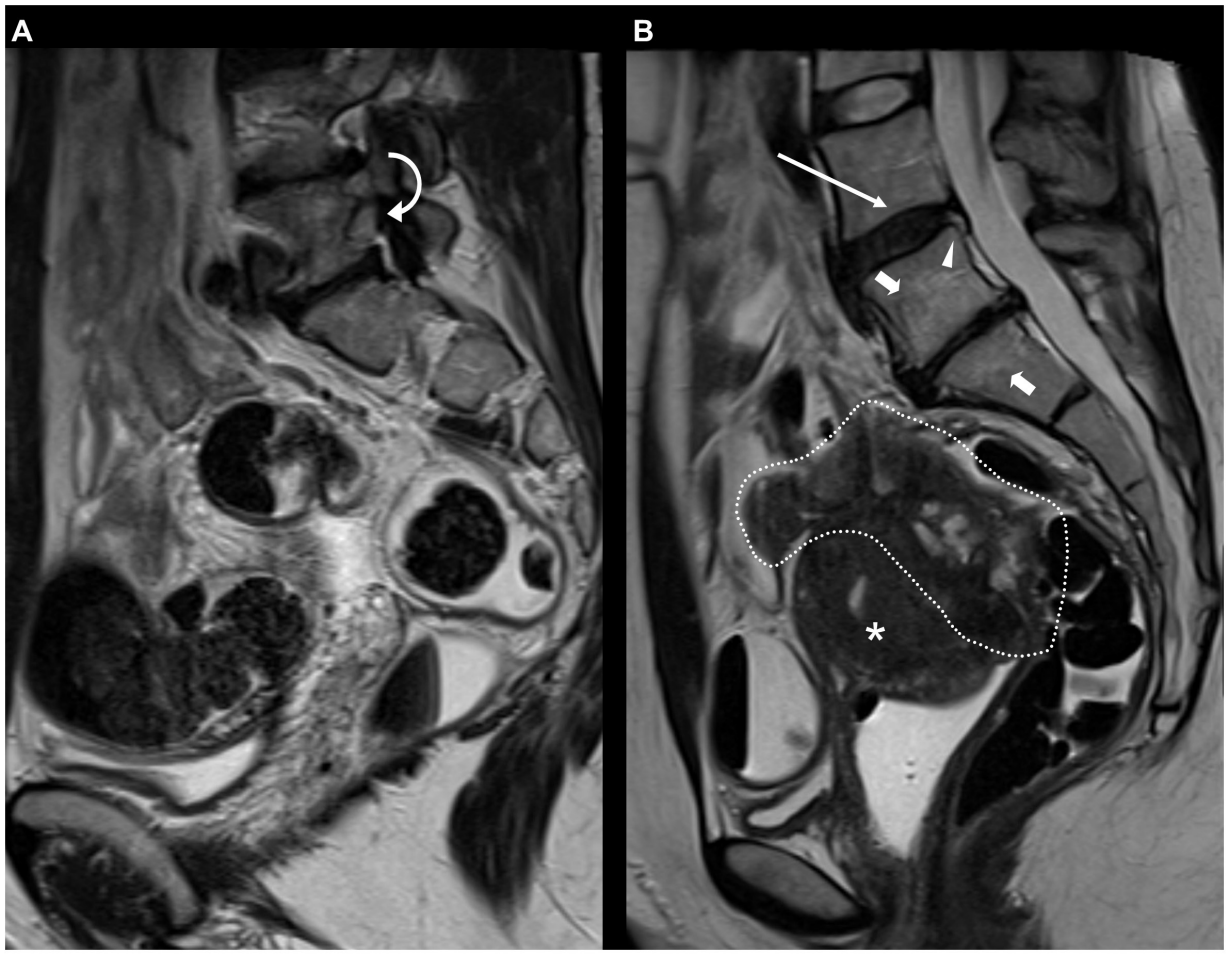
(A) 40-year-old patient with potential foraminal lumbar nerve root compression on MRI for endometriosis, depicted on sagittal T2 FSE (fast spin echo) at L5-S1 level due to decreased height of the intervertebral disc, bulging of the disc and articular process hypertrophy (curved arrow). (B) 36-year-old patient with typical deep infiltrating endometriosis (DIE) and degenerations of the lumbar spine on sagittal T2 FSE (fast spin echo). A large, inhomogeneous mass of DIE is evident in the pouch of Douglas (area encircled by dotted line) with involvement of the vaginal vault, the rectum, the sigmoid colon, and the posterior outer myometrium [A2, B3/3, C3, FA, FI according to the #Enzian classification (56)]. Also included on the MRI slice are degenerative changes of the lumbar spine with desiccation (long arrow) and annular fissure (arrowhead) of the L4-L5 disc, decreased height and extrusion of the L5-S1 disc and associated Modic type 2 signal changes (short arrows). Asterisk: Uterus.
